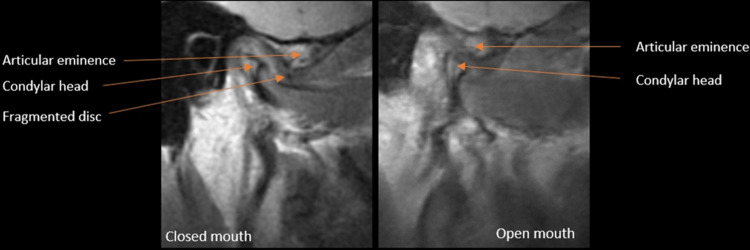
MRI Proton density showing fragmented disc on right side closed mouth view and no translation on the open mouth view
